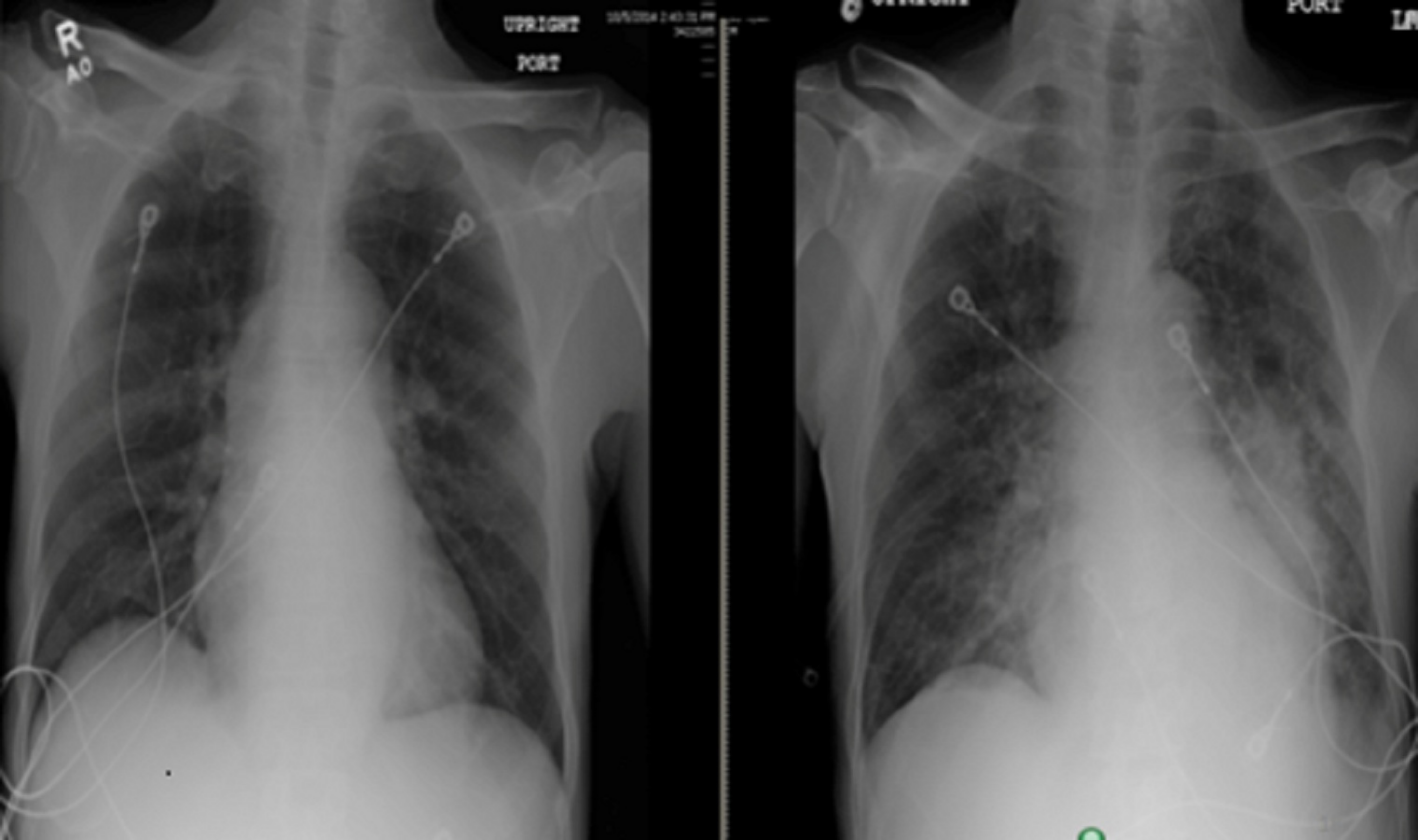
Chest X-ray before (left) and after (right) the surgery showing the development of pulmonary edema after the surgery
